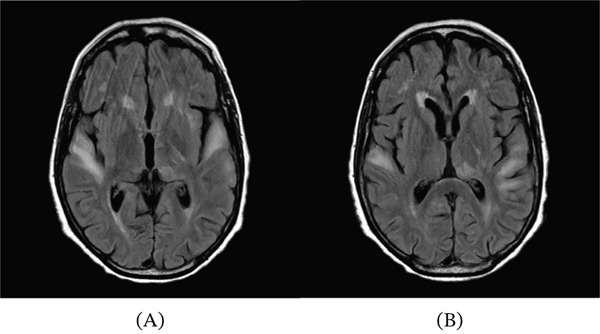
(A, B) Hospital day 2 T2/FLAIR MRI sequences showing new regions of enhancement.
